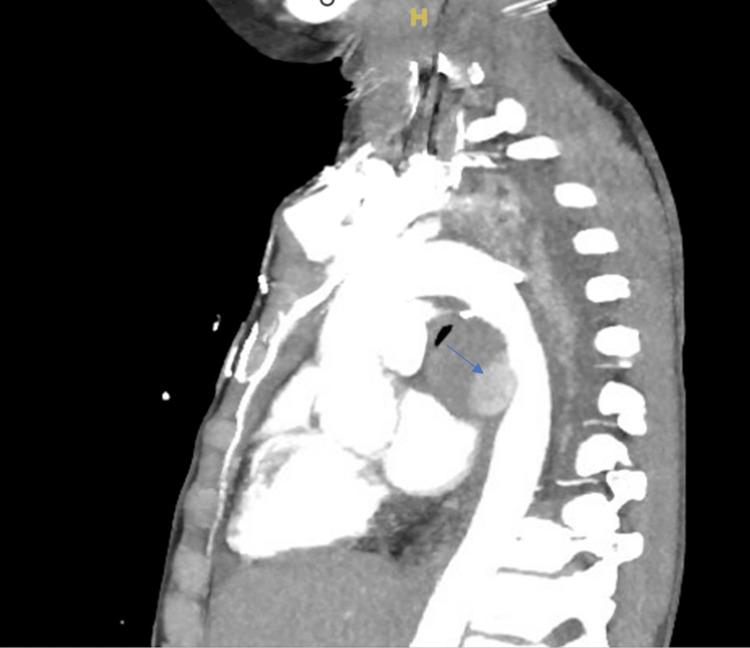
Computed tomography of the chest with contrast The blue arrow shows a pseudoaneurysm of the descending aorta.
